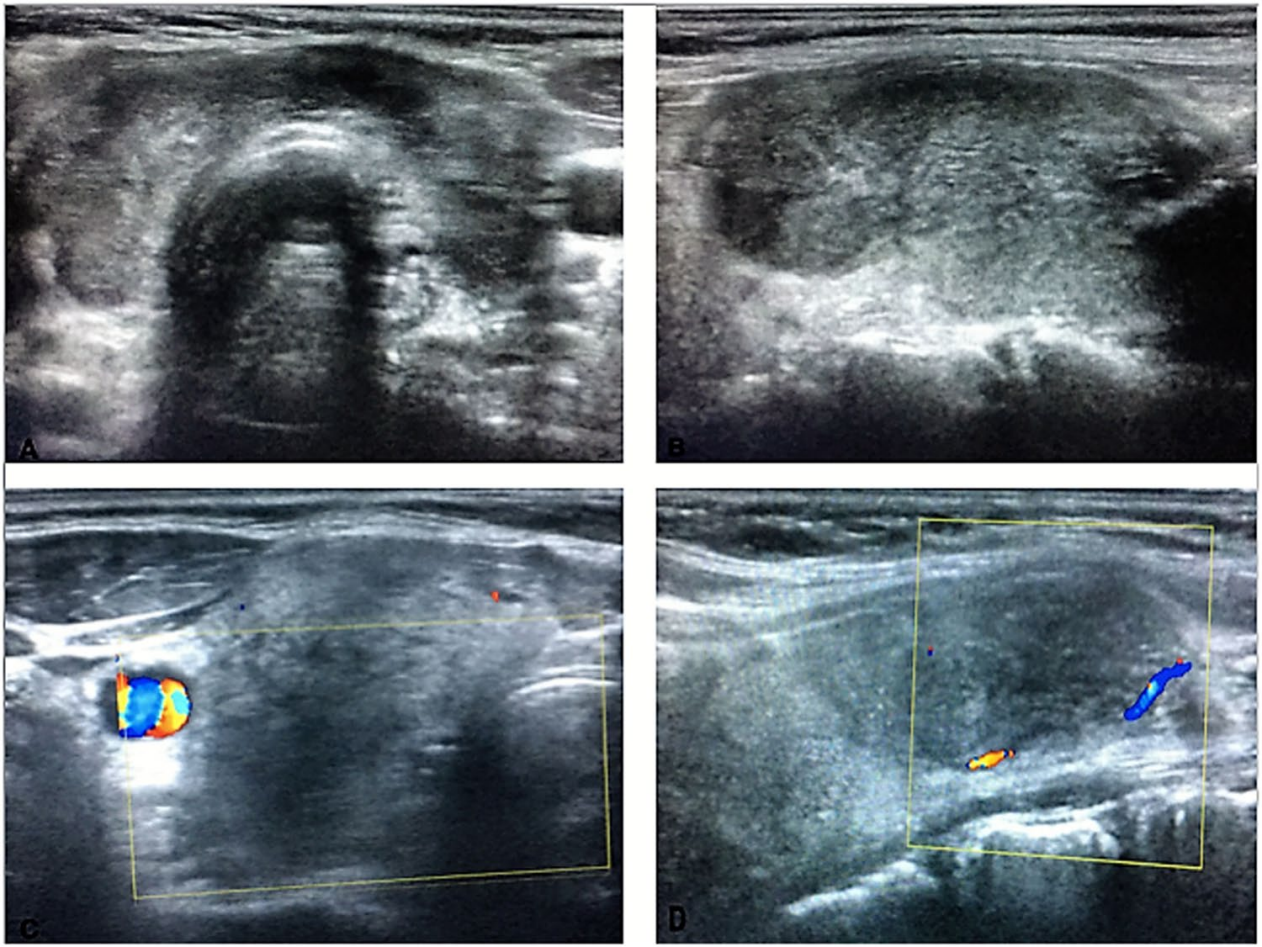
(A) Thyroid ultrasound typically shows a bilateral heterogeneous hypoechoic pattern, (B) hypoechoic heterogeneous area painful to the touch of the probe in the right lobe (C,D) shows no vascular flow in hypoechoic areas.
